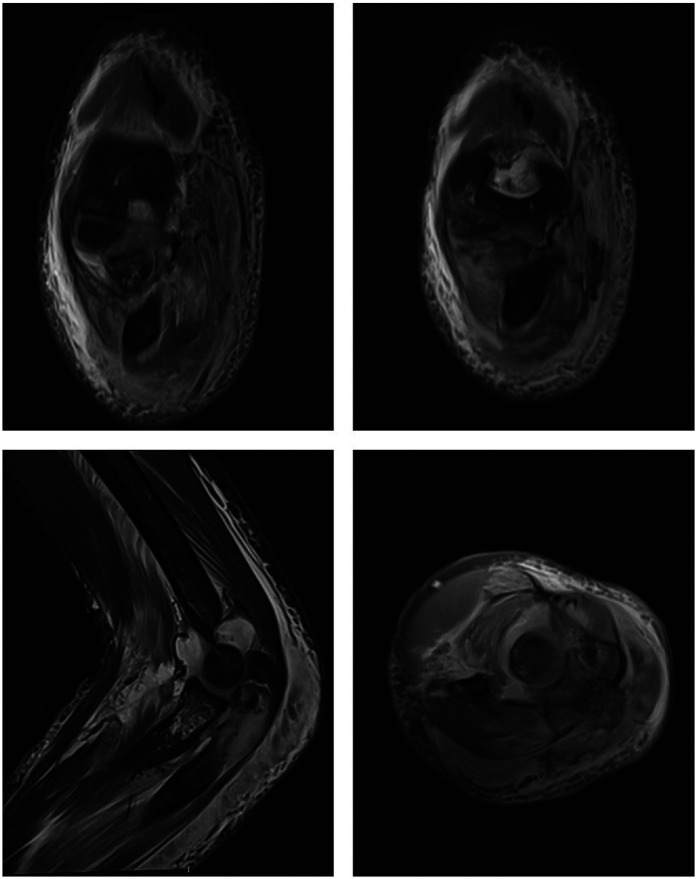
MRI showed tears in the attachment of the brachialis muscle, anterior joint capsule, and anterior periosteum of the proximal ulna, while the ligaments and soft tissues attached to the medial and lateral epicondyles of the humerus remained intact.
